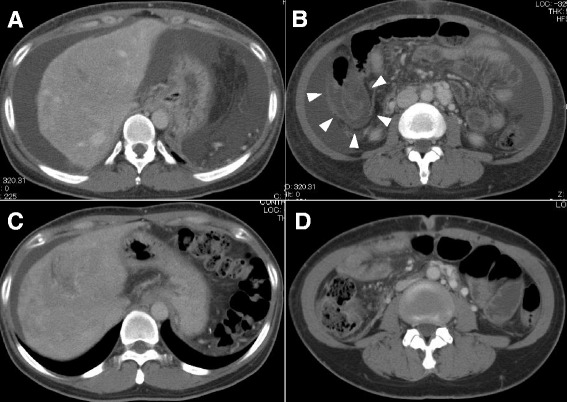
Computed tomography (CT) at the onset of spontaneous bacterial peritonitis and after discharge. a, b CT images obtained at admission. Ascites (a) and remarkably thickened large intestinal wall (*arrowhead*) were confirmed. c, d CT images obtained 1 1/2 month after discharge. Ascites had decreased (c) and the wall thickness of the large intestine appeared normal (d)
